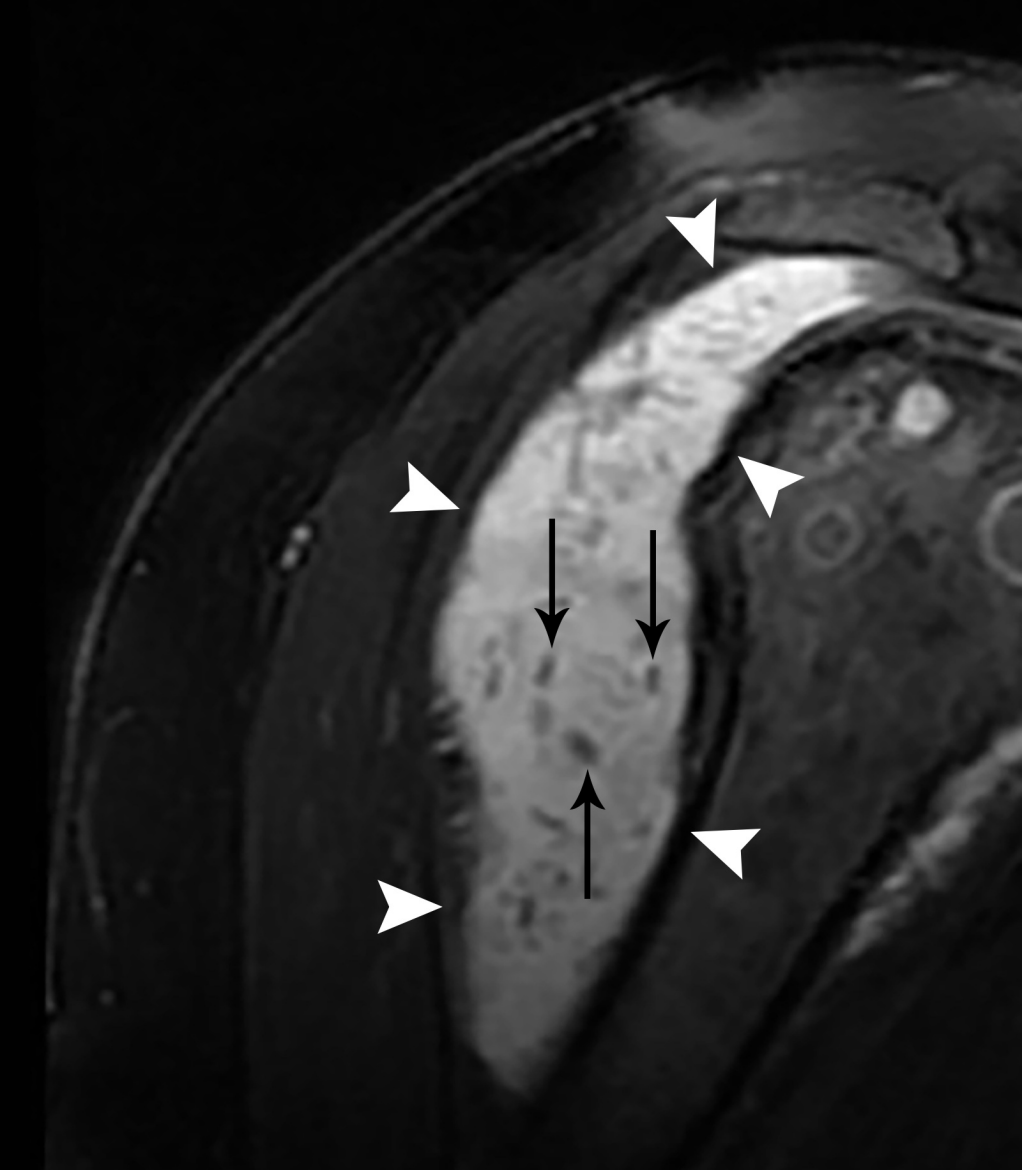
Magnetic Resonance Imaging (MRI) Imaging of a Shoulder Mass in a 28-Year-Old Female. A T2 fat-saturated sequence demonstrates a 10.6 cm hyperintense fluid collection (white arrowheads) with multiple foci of internal debris, consistent with rice body formation (black arrows).
